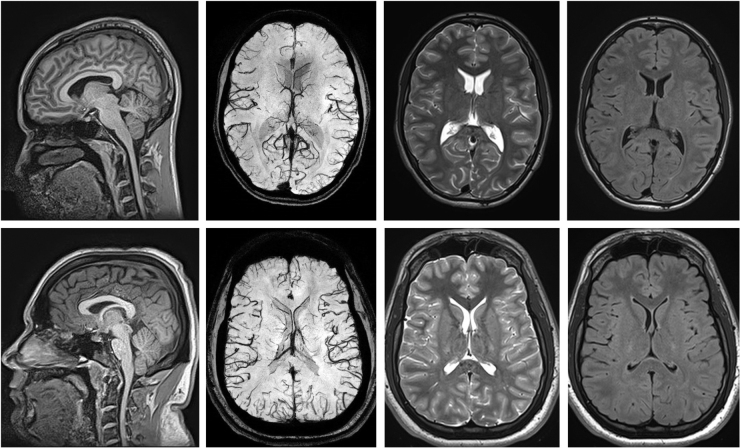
Sample brain images from a player without (top row) and another player with (bottom row) concussion history. The images from left to right are 3D-T1w, 3D-FSBB, 3D-T2w, and 3D-FLAIR. All images are read as normal. 3D, three-dimensional; FLAIR, fluid-attenuated inversion recovery; FSBB, flow sensitive black blood; T1w, T1-weighted; T2w, T2-weighted.
